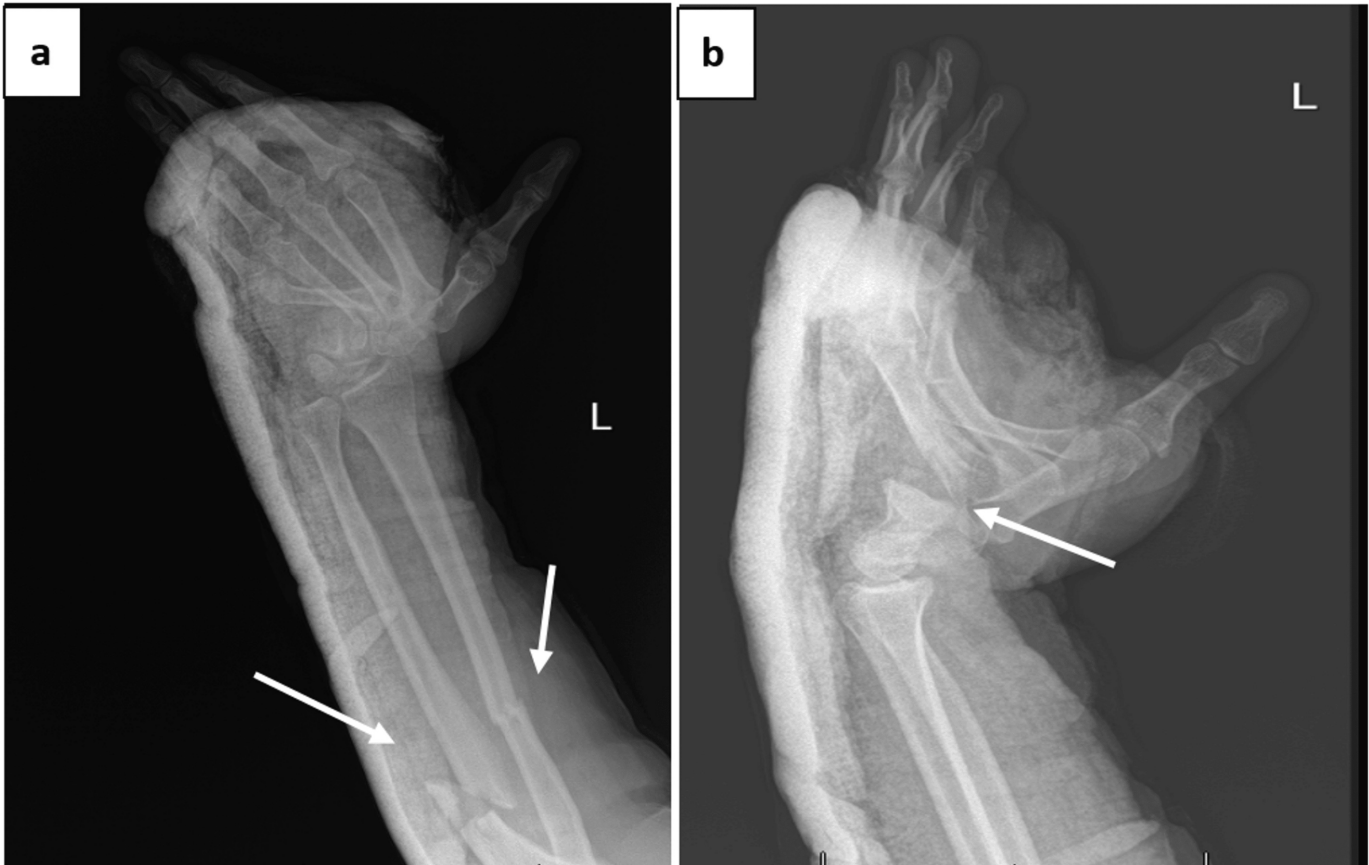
Additional X-ray views confirming left carpometacarpal (CMC) joint dislocation: (a) oblique view, (b) lateral view highlighting dorsal displacement
